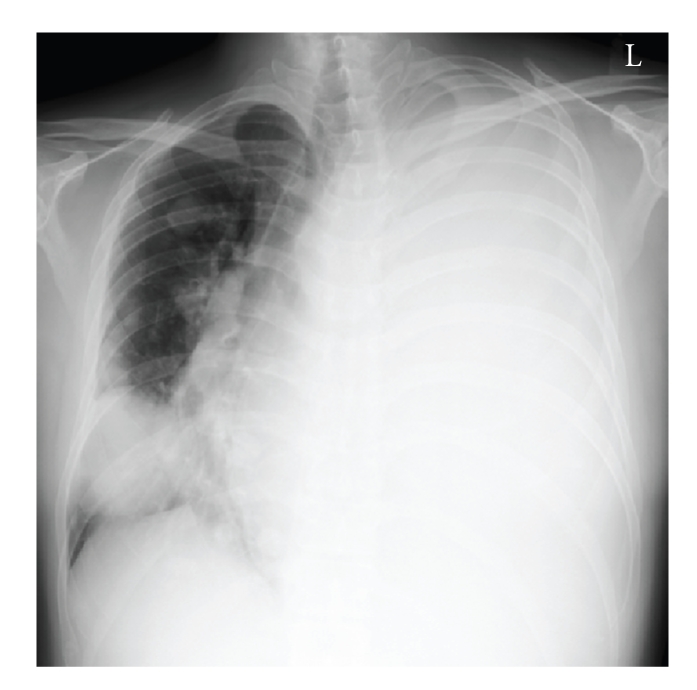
Plain radiography of lung at the final follow-up. Secondary lesion in left lung grew remarkably, and mediastinum was pressured severely.
